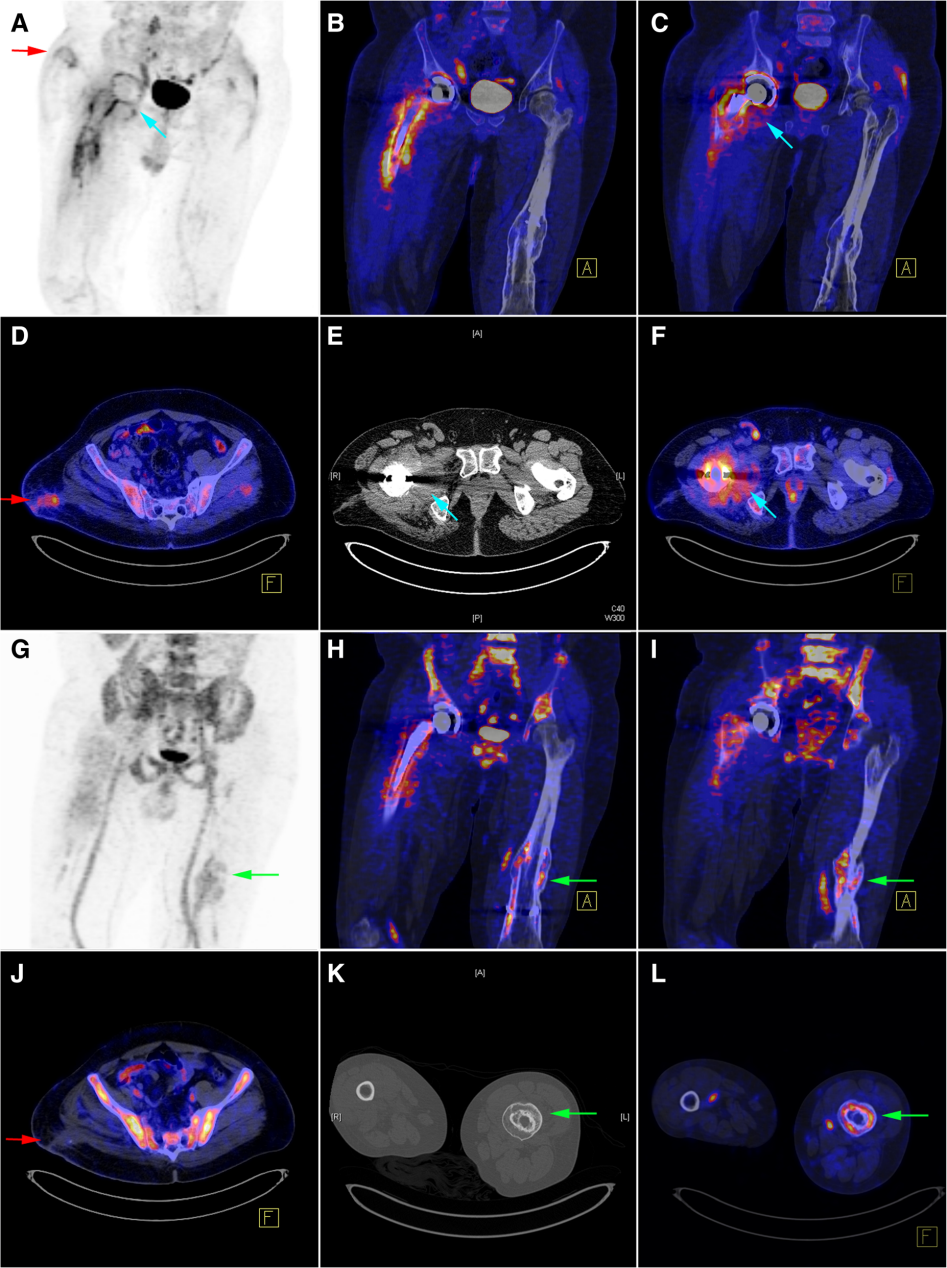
Patient (case # 1) with a history of right hip prosthesis implantation performed 7 months before imaging. The results of ^18^F-FDG PET/CT (upper two rows) and ^68^Ga-citrate PET/CT (lower two rows) are presented. a–f The red arrows clearly indicate ^18^F-FDG uptake occurring at the sinus tract, which was absent on the corresponding ^68^Ga-citrate PET/CT image. There was also evidence of swollen periarticular soft tissue (blue arrows) at the right ischiofemoral space on CT, which was accompanied by an increased ^18^F-FDG uptake. A similar pattern of radiotracer uptake (b, h) was found to occur at the bone-prosthesis interface of the stem component, as well as in the adjacent soft tissue. The imaging results were considered positive, and the case was subsequently classified as true positive. g–l The green arrows indicate an increased ^68^Ga-citrate uptake occurring at the left femoral shaft. The CT scan revealed cortical hypertrophy caused by a prior traumatic fracture followed by bone reunion
